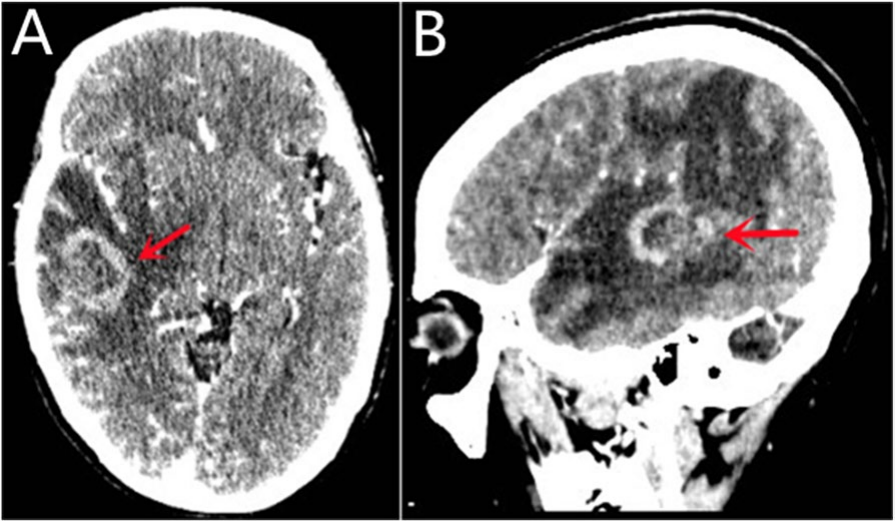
Brain MRI showed an irregular mass in the right temporal lobe, with obvious uneven enhancement on the enhanced scan, and a large area of non-enhancing edema around it, involving the basal ganglia in the (A) sagittal plane and (B) transverse plane
